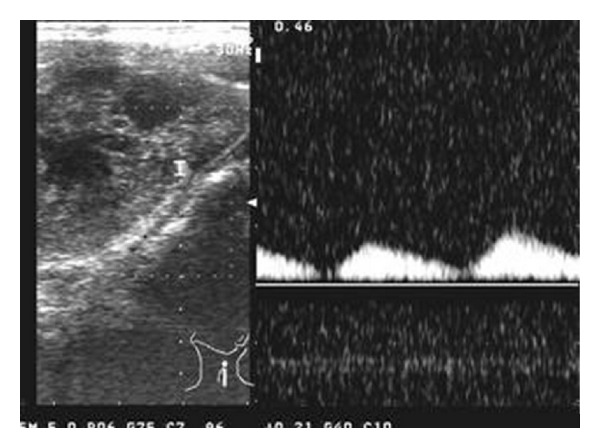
The Doppler ultrasonography of neck reveals a heterogeneous predominantly hypoechoic mass on the left carotid space showing mild peripheral vascularity.
